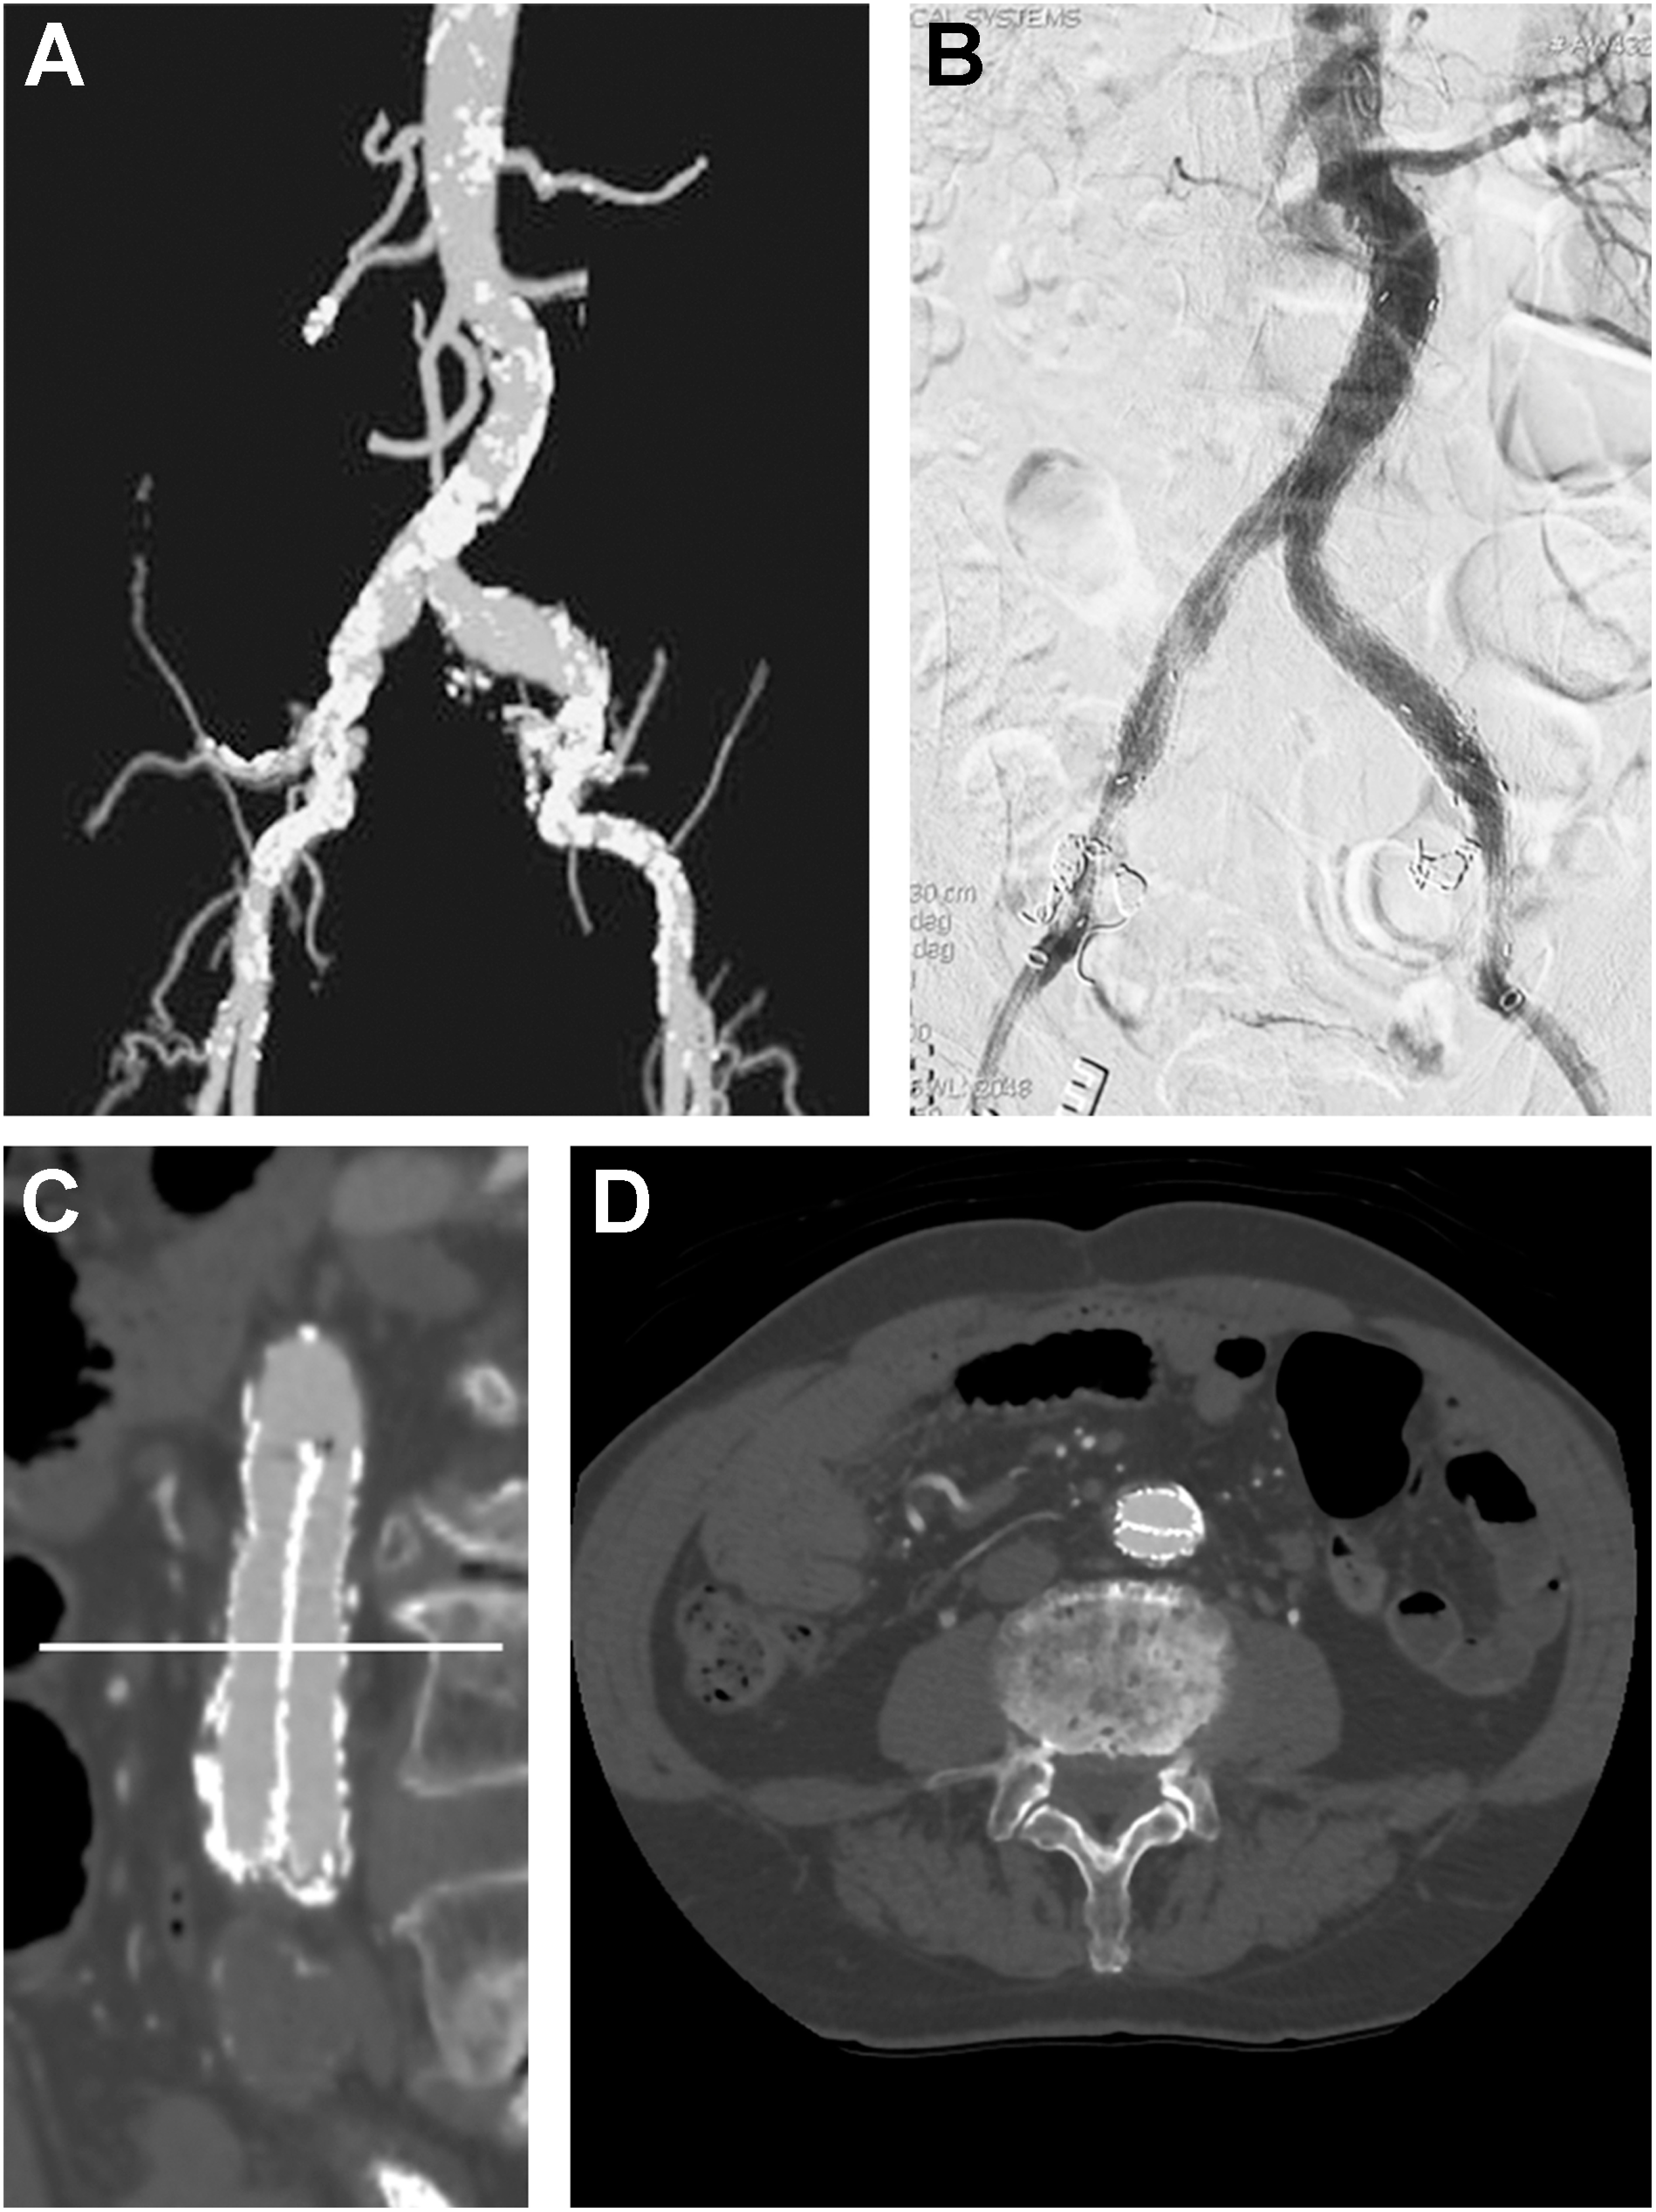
Fig. 1 (A) Maximum intensity projection image of preoperative contrast-enhanced computed tomography (CT) shows marked calcification of both external iliac arteries. (B) Completion angiogram shows no endoleak after parallel placement of Excluder legs. (C) The multi-planar reconstruction image of postoperative CT shows parallel configuration of Gore Excluder legs inside the abdominal aorta. The white horizontal line shows the level of the axial image (D). (D) The axial image shows complete sealing and no collapse of legs.
